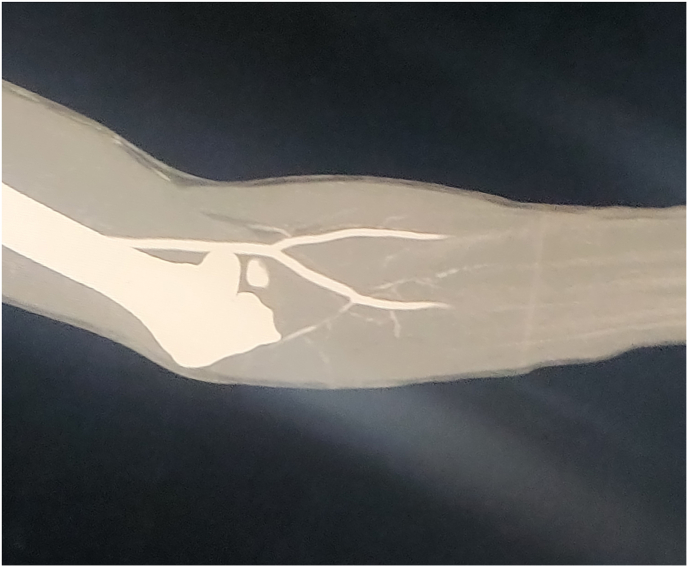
Computed Tomography Angiography image of left upper limb showing non opacification of radial and ulnar artery about 3 cm distal to their origin.
